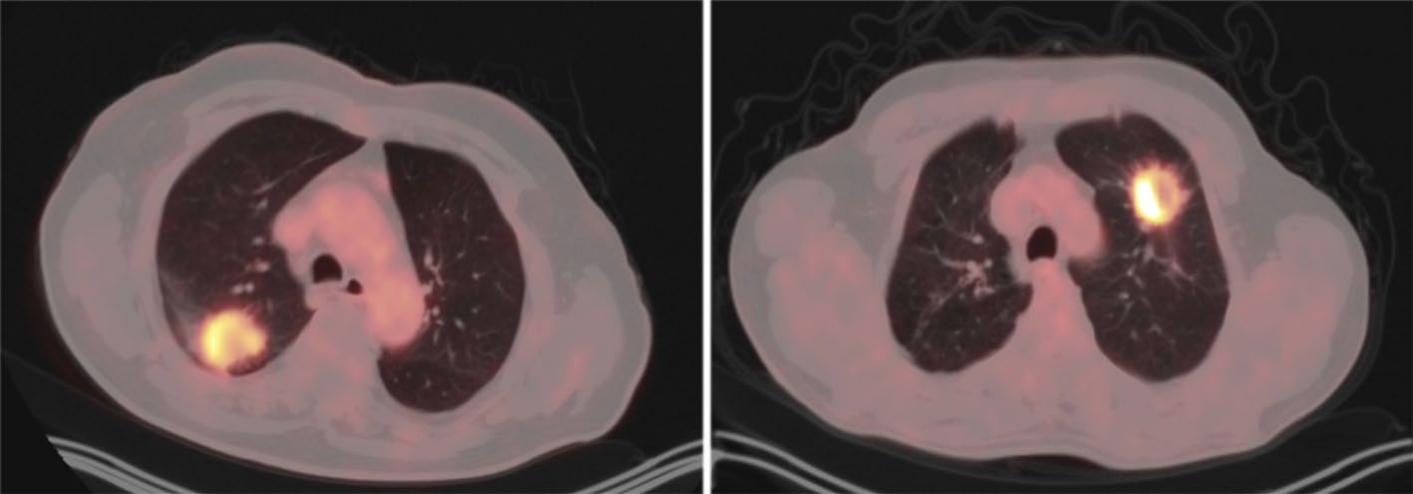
Representative pictures of ADC (left) and SCC (right). SUVmax values for ADC and SCC are 13.03 and 21.22, respectively
